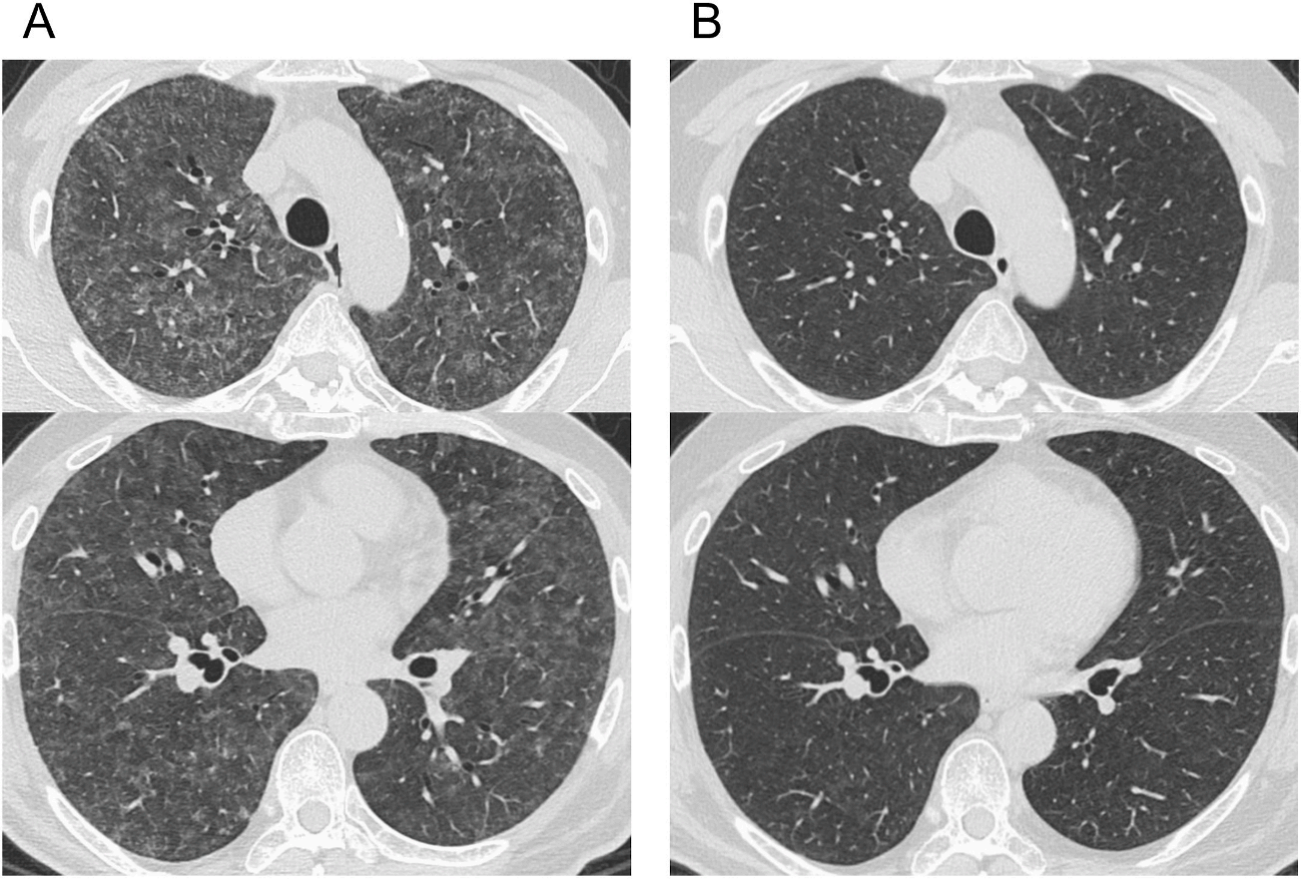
High-resolution computed tomography (HRCT) images of the lung. (A) HRCT on admission showing diffuse ground-glass opacities with ill-defined centrilobular nodules throughout both lungs with upper lobe predominance. (B) Complete resolution of the abnormalities after 3 months of steroid treatment and discontinuation of the whirlpool bath.
